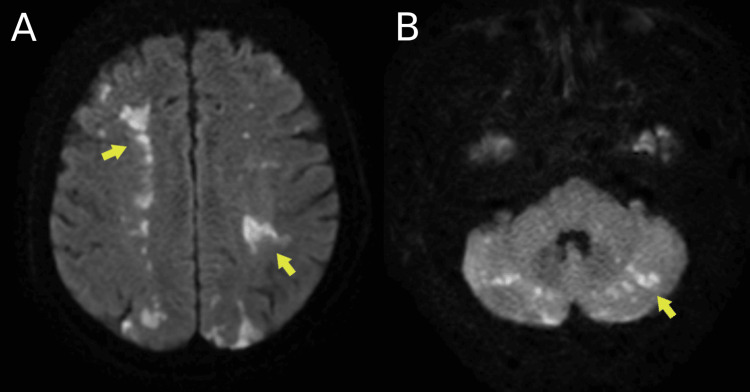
MRI brain (DWI) on day six Diffusion-weighted imaging (DWI) demonstrates hyperintense lesions (yellow arrows), indicating acute infarcts in bilateral watershed territories. Infarcts are visible in (A) the frontoparietal lobes and (B) cerebellum, consistent with multifocal ischaemic injury.
